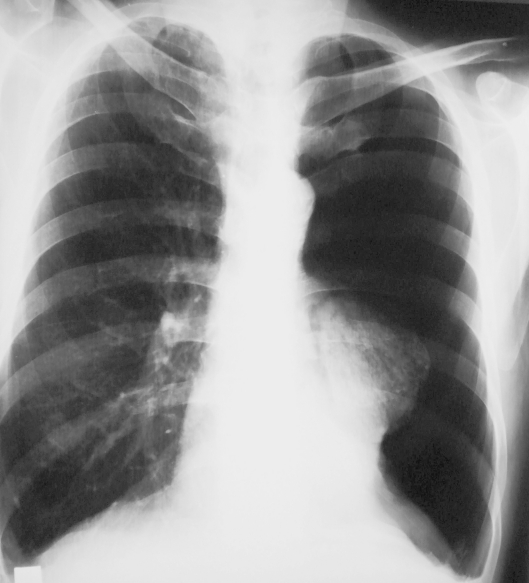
Chest x-ray showing an air density in left lung fields
